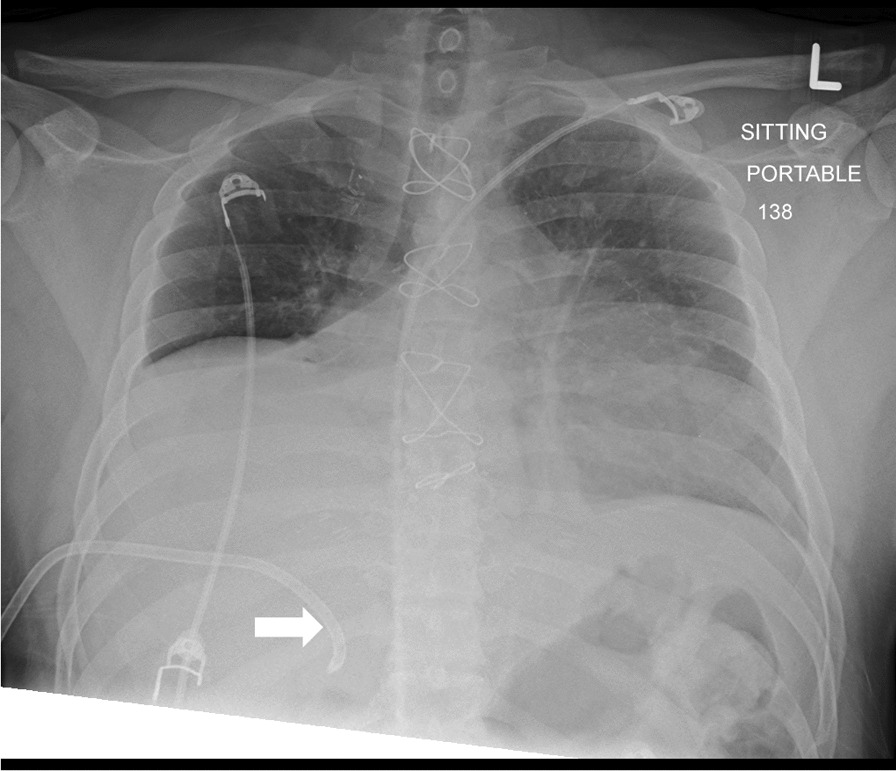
The post-pleural drainage catheter insertion portable sitting chest X-ray demonstrates the presence of a right-sided pleural drainage catheter (white arrow) passing through the 7th intercostal space, with its tip positioned over the liver shadow. Unfortunately, the primary physician misinterpreted this as a right-sided large pleural effusion
